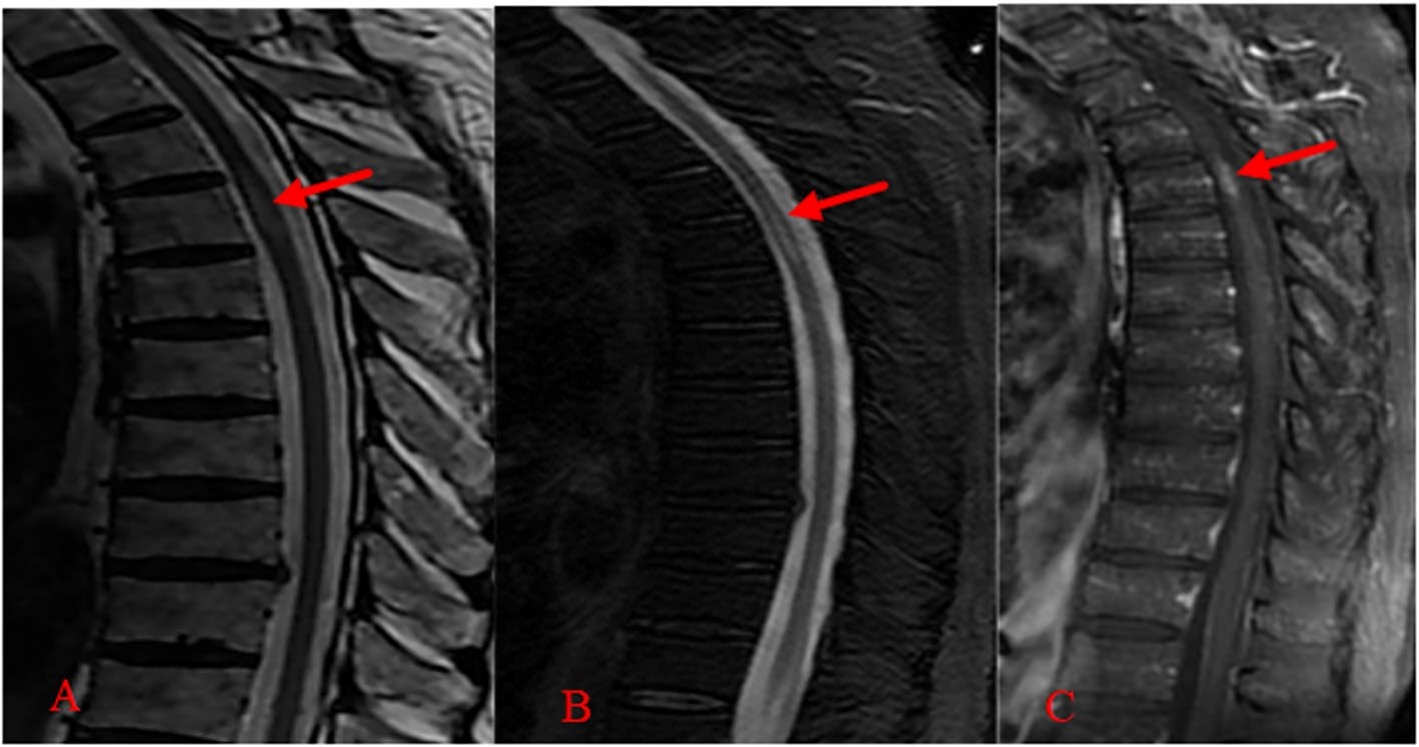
Contrast-enhanced MRI scan of thoracic vertebrae (2020–08-17). abnormal signal in the spinal cord at T5 vertebra level,Swelling of T4-6 spinal cord on T2 Flair (A) and T2 (B), with local enhancement (C)
